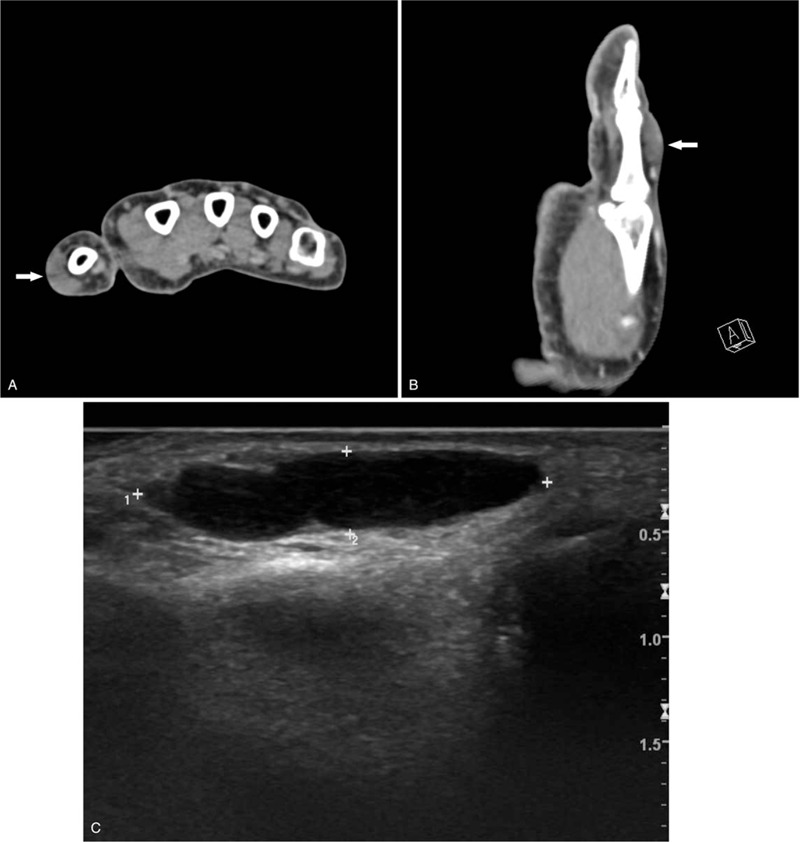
Preoperative CT and US images. (A), (B) Preoperative CT images. (C) Preoperative US image. CT = computed tomography, US = ultrasonography.
